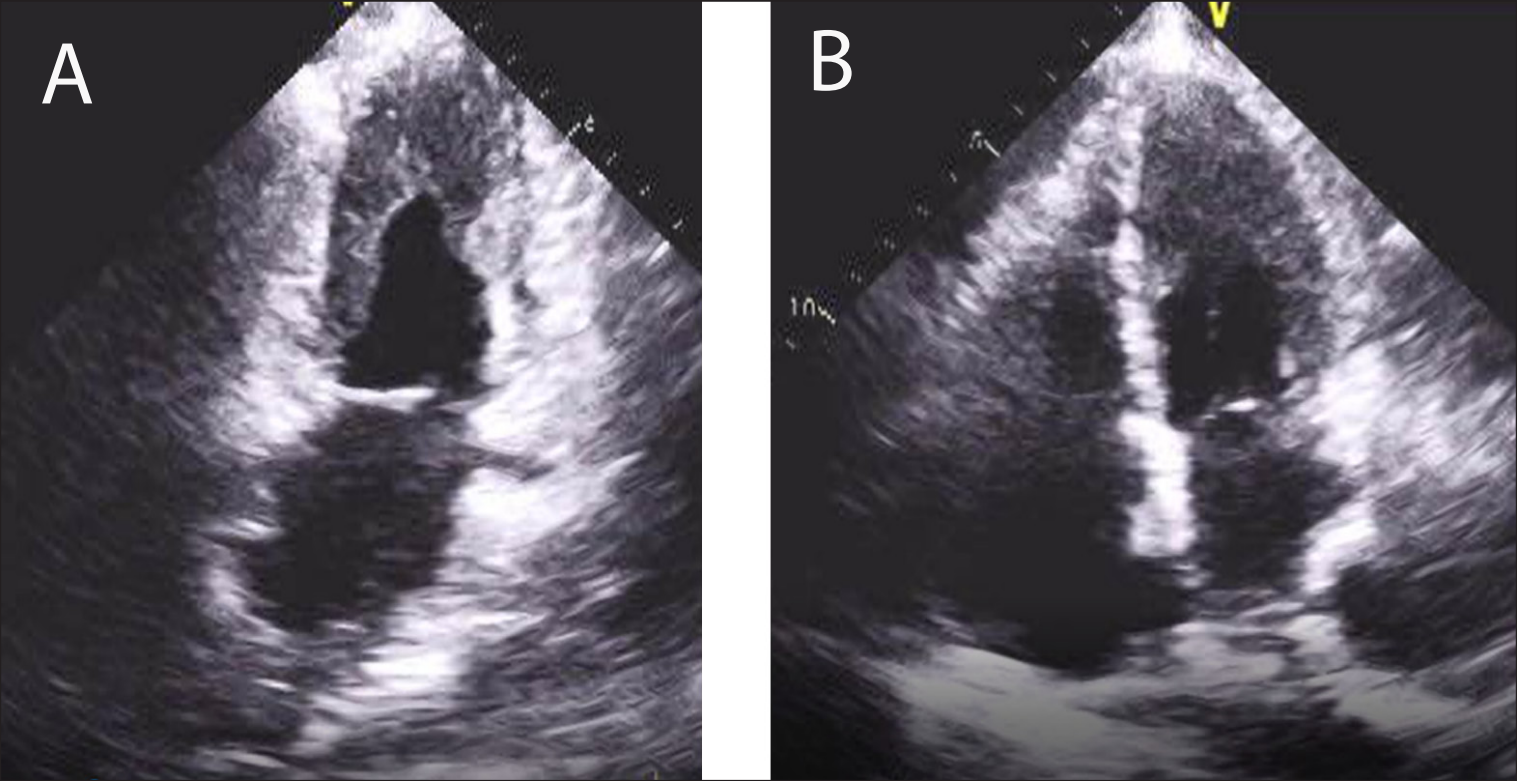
Apical 2-chamber and 4-chamber echocardiographic view showing biatrial enlargement, apical obliteration by thrombus.
